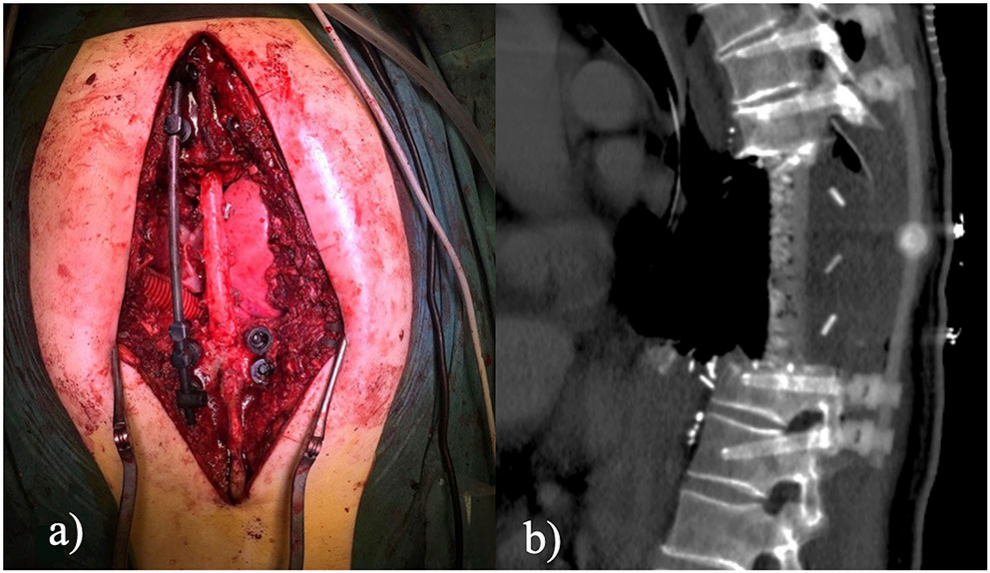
(a) Open posterior approach. The figures show the results of a triple *en bloc* vertebrectomy. Pedicle screws were inserted at two levels above and two levels below the pathologic vertebrae; (b) Sagittal CT scan. The figure shows the final result after tumor removal and 360° fixation. Instrumentation is radiolucent to consent radiologic follow-up.
